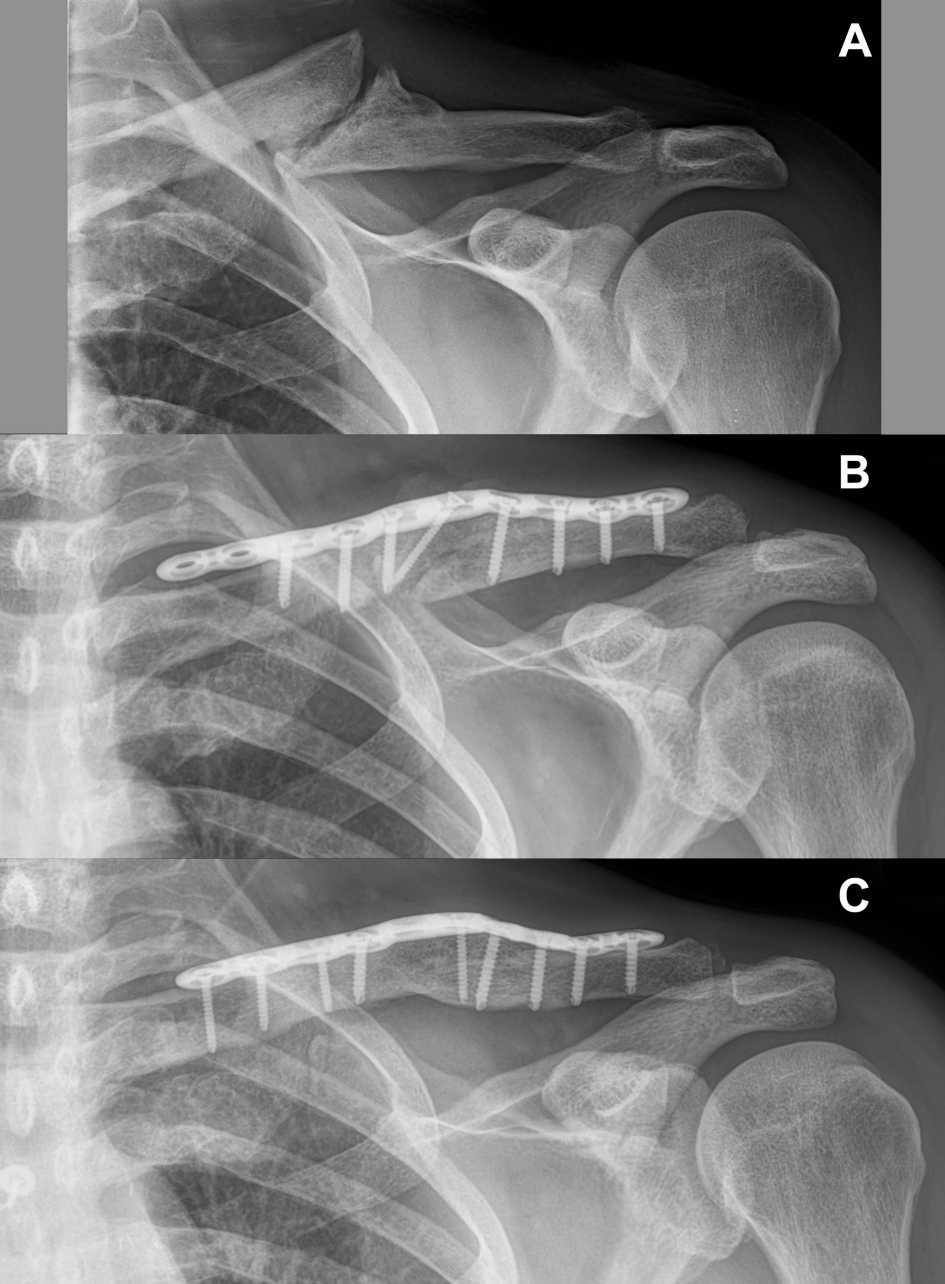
Anterior-to-posterior radiographs of the patient’s left shoulder. These radiographs were taken: (A) in September 2018, two months before the patient’s index clavicle surgery, and show a hypertrophic midshaft clavicle fracture nonunion; (B) in early November 2018, and show the hardware after the index clavicle reconstruction surgery, which resulted in some clavicle lengthening; and (C) in 2024, and show the state of the hardware after the revision surgery (performed in mid-November 2018), when additional clavicle lengthening occurred because the fracture was gapped open further and the metal plate was bowed upward. The clavicle fracture is well healed.
